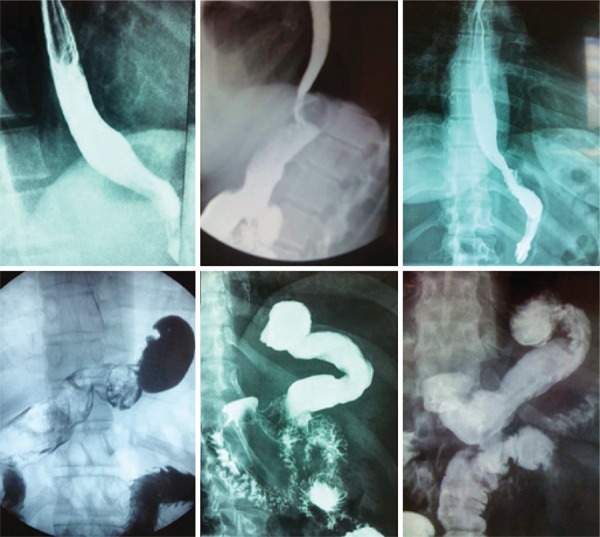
Upper GI radiographies with barium swallow after ARSG. Three top images show patients in upright position. Three images at the bottom show patients in a Trendelemburg position (it is observed that contrast accumulates at the top of the stomach) and Valsava maneuver (the stomach is compressed against the diaphragm). No esophageal reflux was seen even under adverse conditions. The inferior right image is ARSG+TB: observe the latero-lateral gastroileal anastomosis in the antrum
